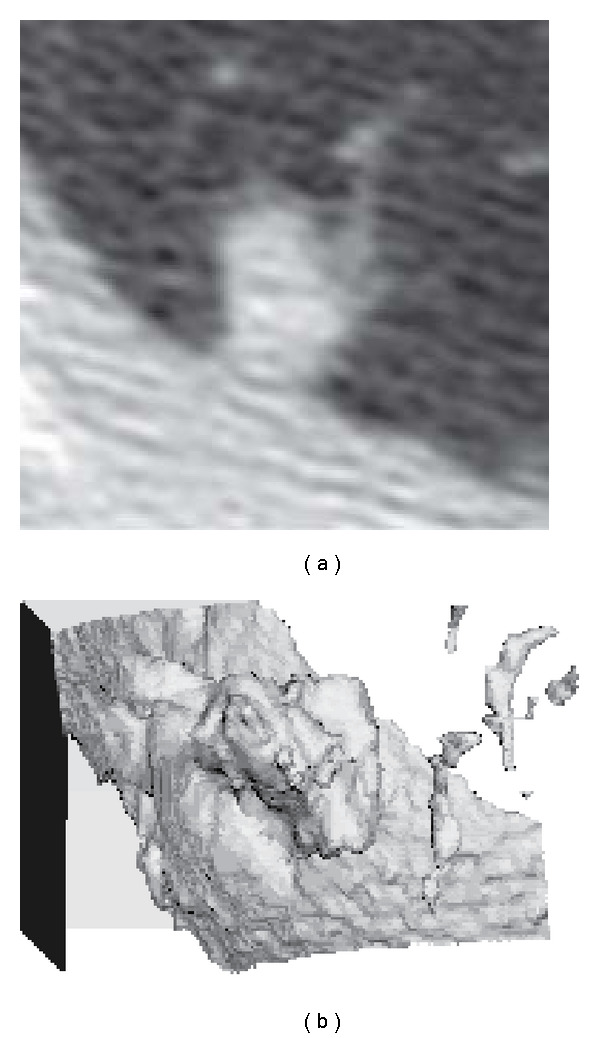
Example of a juxtapleural nodule, with (a) one central slice from CT scan and (b) 3D visualization of region of interest.
